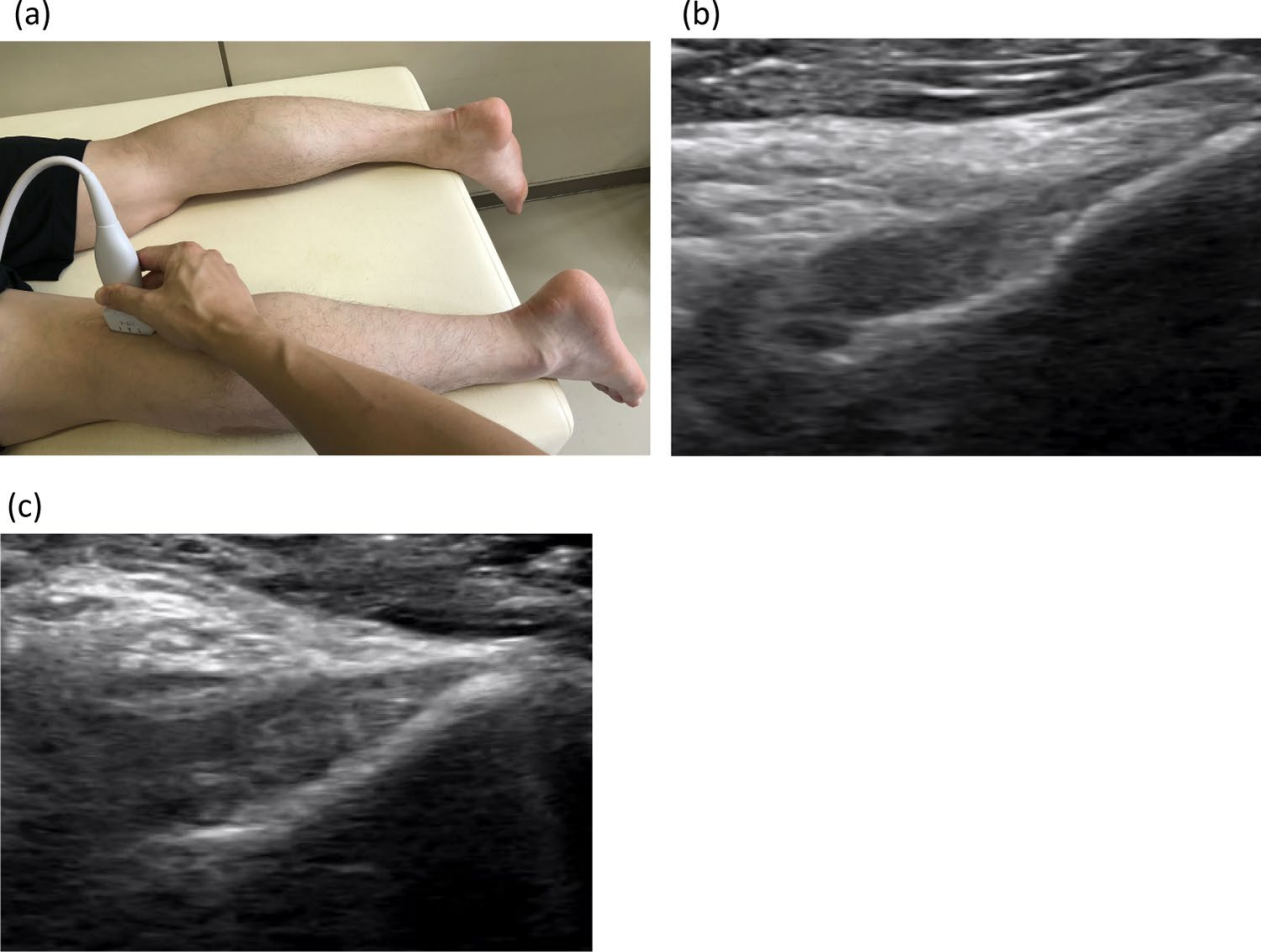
Ultrasonographic delineation of the posterior cruciate ligament. a The patient was placed in the supine position with the knee in full extension, and the probe was placed in the popliteal region, longitudinally from the intercondylar area of the proximal tibia. b An ultrasound image of the same patient as in Fig. 1; on the image of the healthy side, the posterior cruciate ligament is depicted as a low-echoing fan-shaped structure tapering distally in the proximal tibia. c Ultrasound image of the affected side showing a thicker posterior cruciate ligament compared to the healthy side
